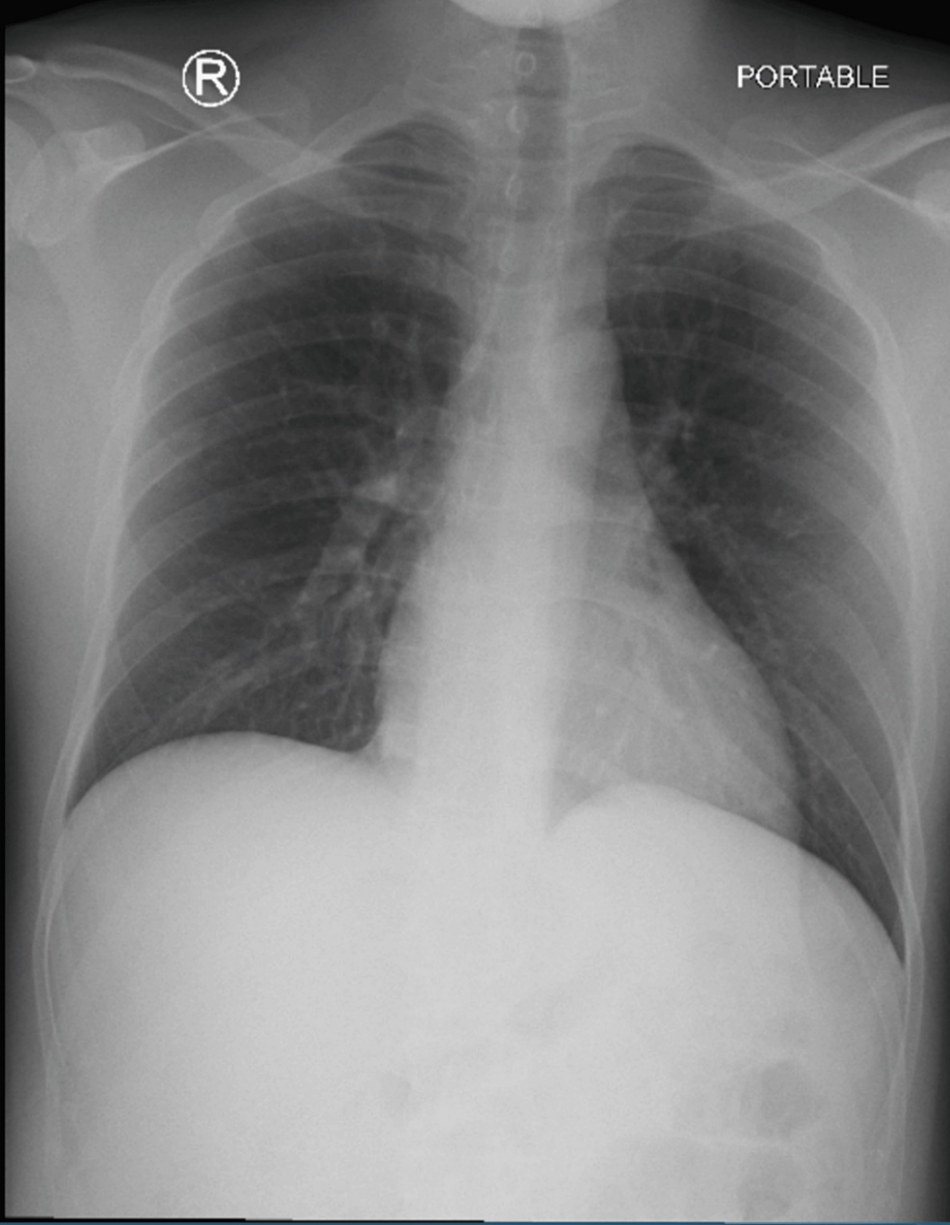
Chest X-ray anterio-posterior view. The cardiomediastinal silhouette is within normal limits in size
